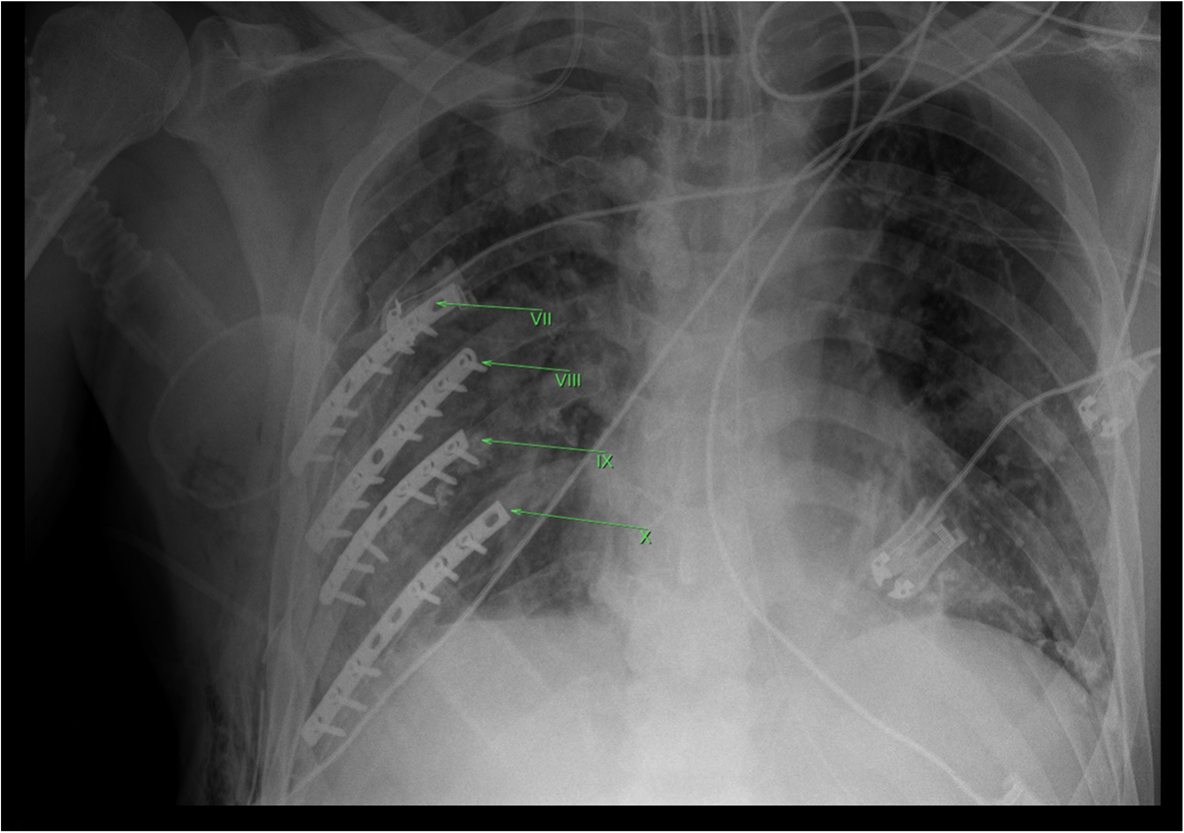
Postoperative chest x-ray of a patient with multiple rib fractures treated via thoracotomy and plate fixation. X-ray image displays the correct placement of the reconstruction plates and cortical locking screws on ribs 7–10
